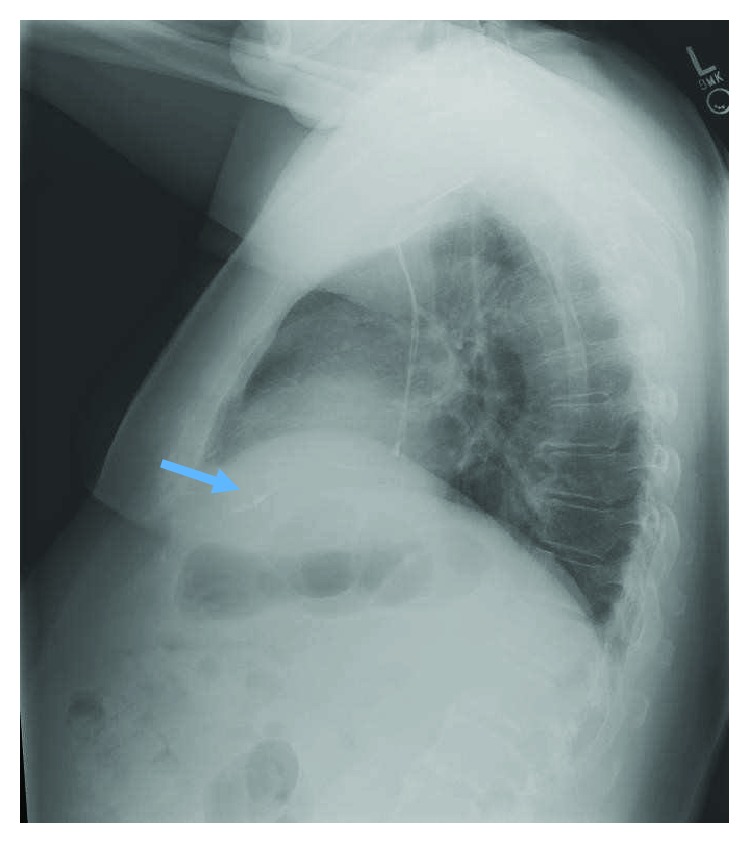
Lateral chest X-ray showed right atrial lead fracture (blue arrow).
